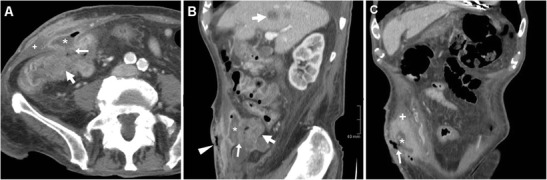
Cutaneous fistulisation of colon carcinoma in a 92-year-old man with worsening right-sided abdominal pain and tenderness. CT detected a segmental, non-stratified circumferential thickening (thick arrows) at the ascending colon, which infiltrated the peritoneum, opened (arrows in A, B) into an abscess (*) in the inflamed abdominal wall (+), and communicated to the skin EFO (arrowhead in B) through an ECF (arrow in C). Liver metastases (thick arrow) were present. The patient was treated supportively with positioning of colostomy-type bag at the EFO (Partially reproduced from Open Access ref. no [35])
